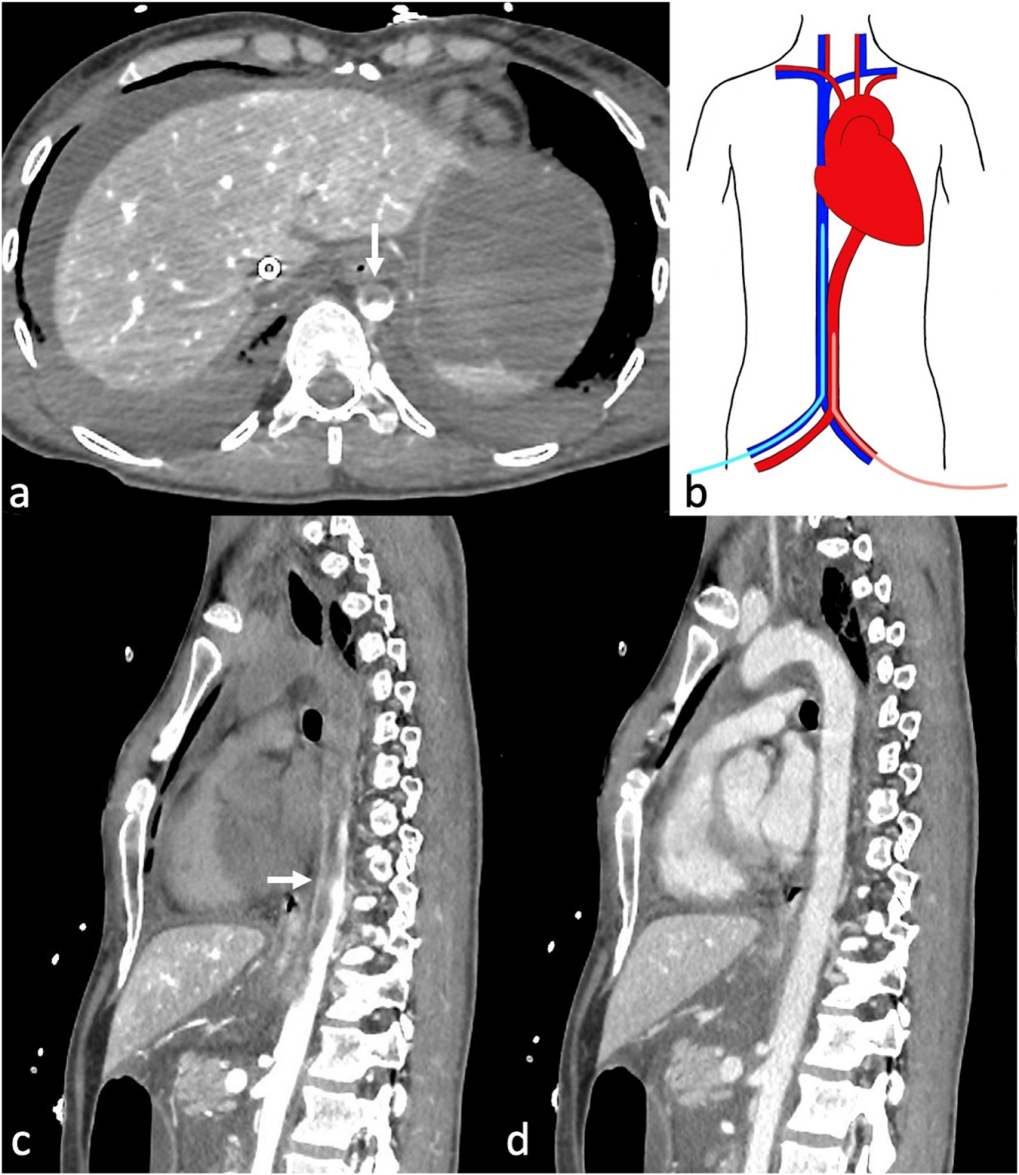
Axial (a) and sagittal (c) computed tomography (CT) images in the arterial phase of a veno-arterial (VA) extracorporeal membrane oxygenation (ECMO) supported patient with classic femoro-femoral configuration (b), showing the “arterial pseudomembrane” phenomenon. This occurs in severe heart failure cases, where enhanced blood from the femoral artery meets the low-enhanced blood from the heart. In such instances, a delayed phase (d) is required to prevent the misdiagnosis of an aortic dissection
